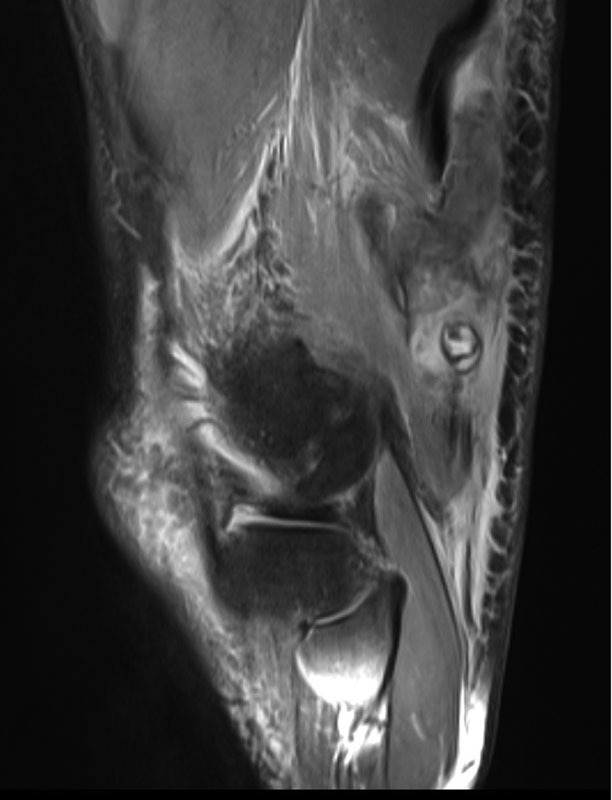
Magnetic resonance imaging finding.
